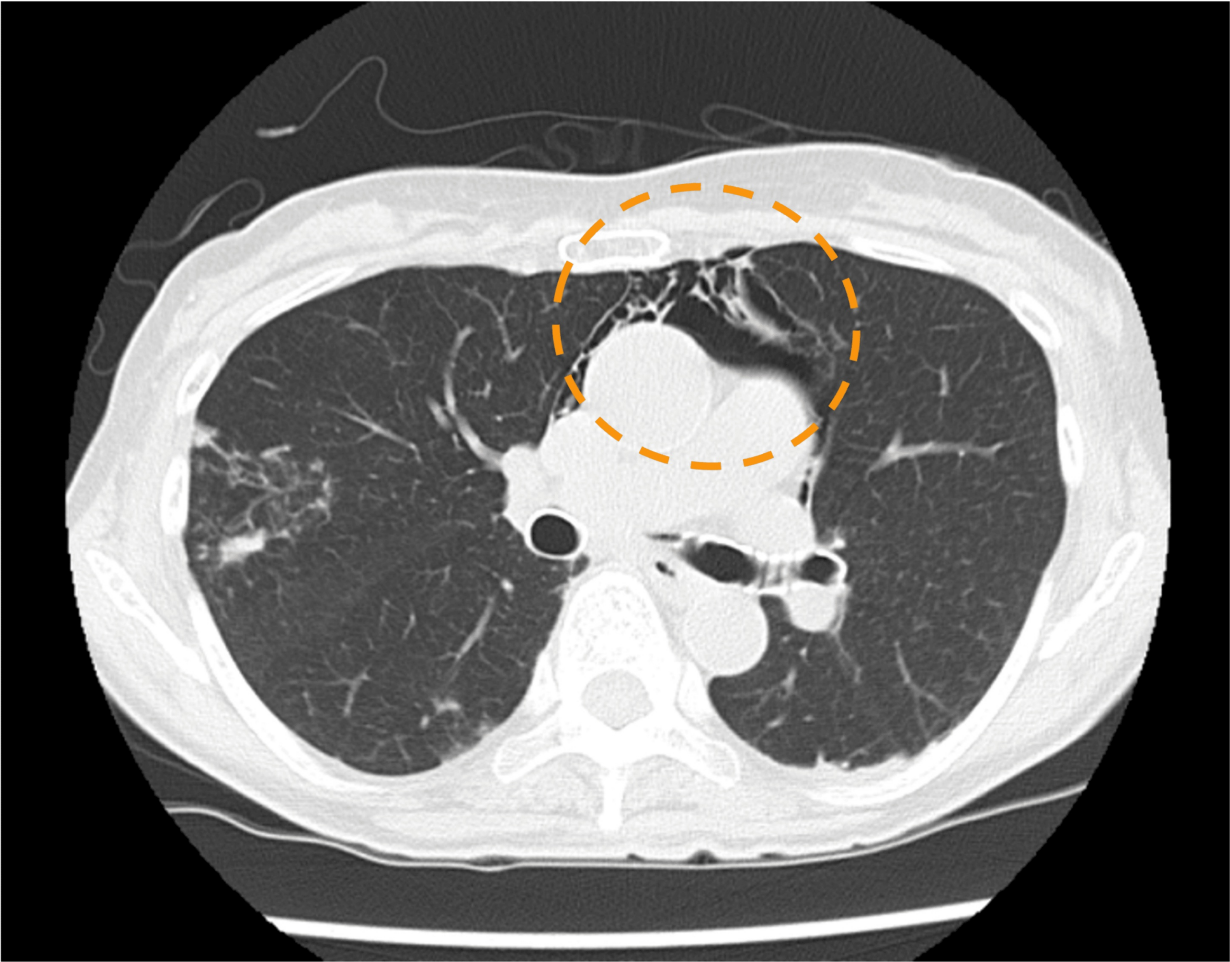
Postoperative CT image showing the presence of free air in the mediastinum and right cervical region CT: computed tomography
